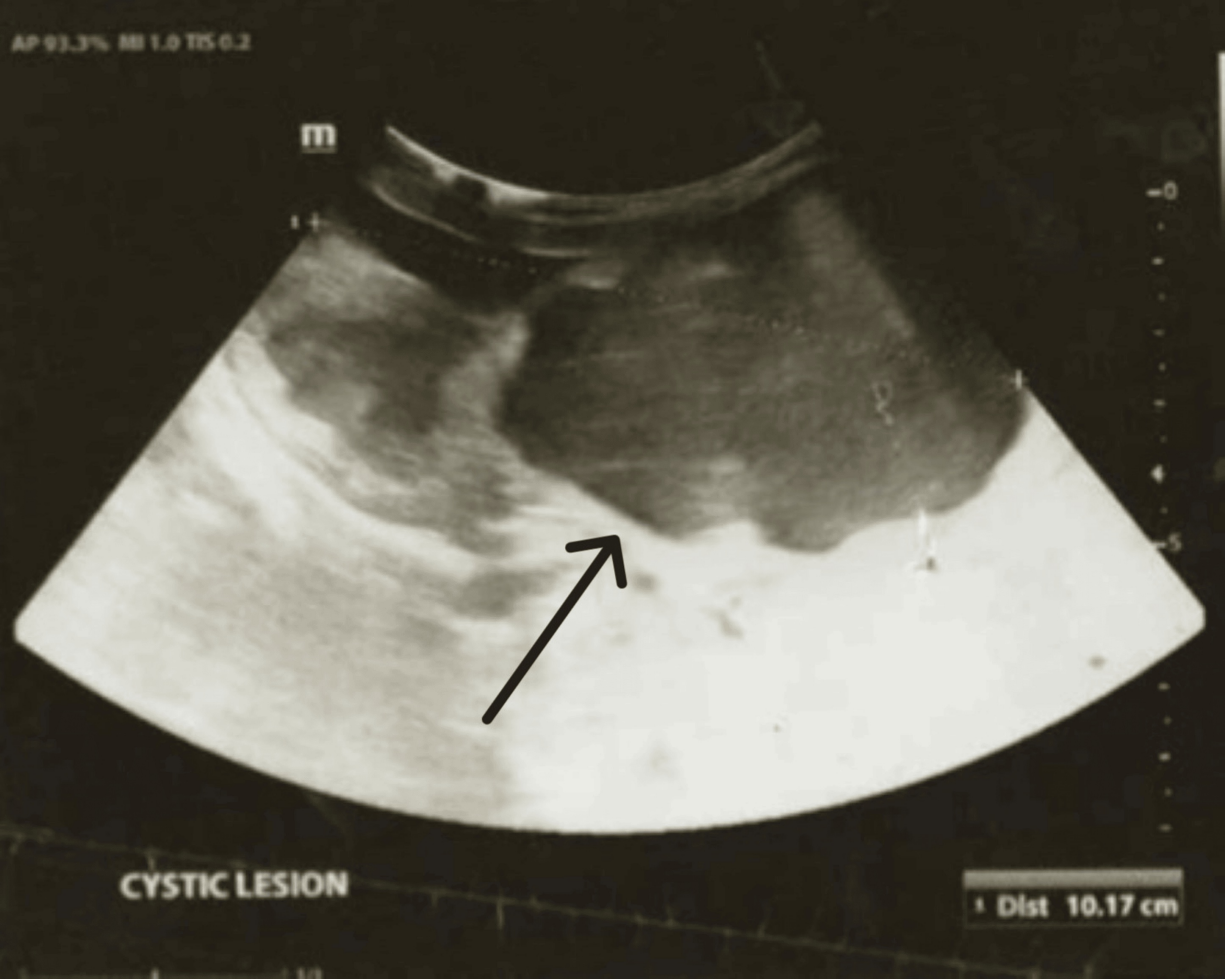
Ultrasound showing cystic lesion in region of right kidney (black arrow)
